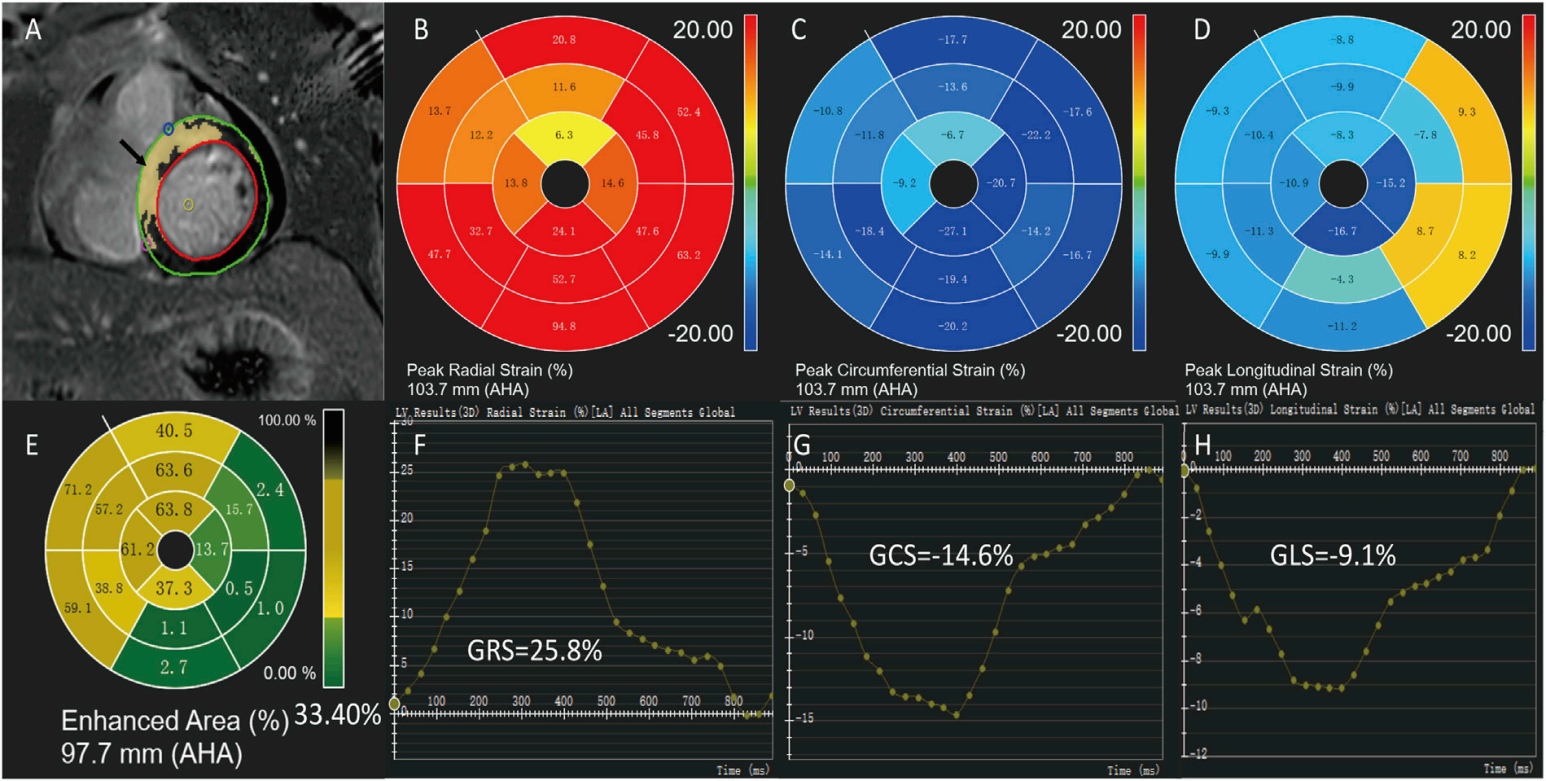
Measurement of cardiac magnetic resonance imaging. A 43-year-old male of ST-segment elevation myocardial infarction with preserved left ventricular ejection fraction. Epicardial (green) and endocardial (red) contours, LGE areas are marked in yellow (A,E). Feature-tracking strain values of the same patient show global radial strain (GRS) = 25.8% (B,F), global circumferential strain (GCS) = -14.6% (C,G) and global longitudinal strain (GLS) = -9.1% (D,H).
